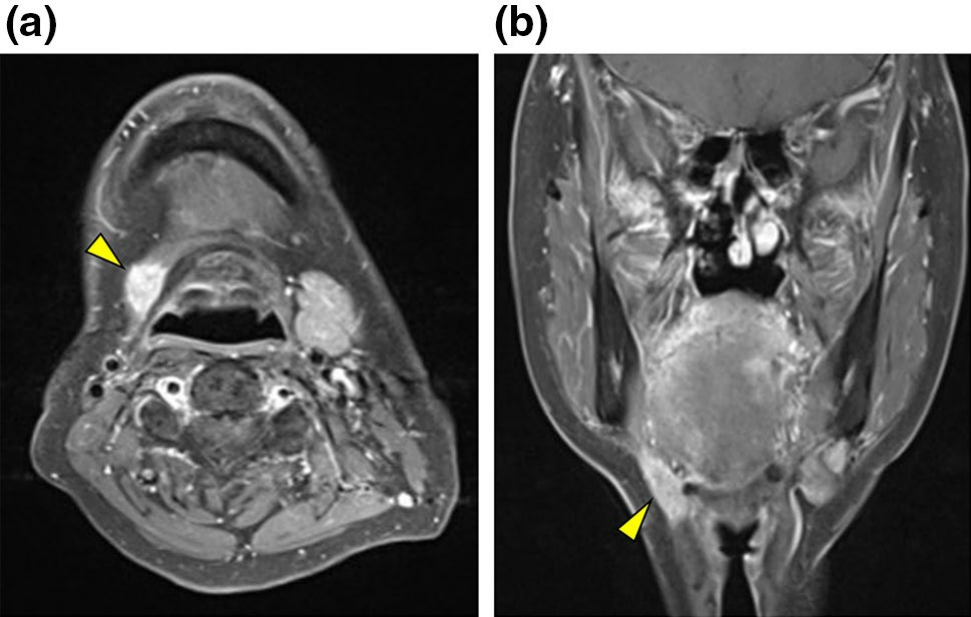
Imaging of lateral lingual lymph node metastasis (LLNM) in the parahyoid area. Lateral LLNM (arrowhead) is demonstrated in the parahyoid area on the right side on (a) enhanced axial T1-weighted magnetic resonance (MR) and (b) enhanced coronal T1-weighted MR images.
